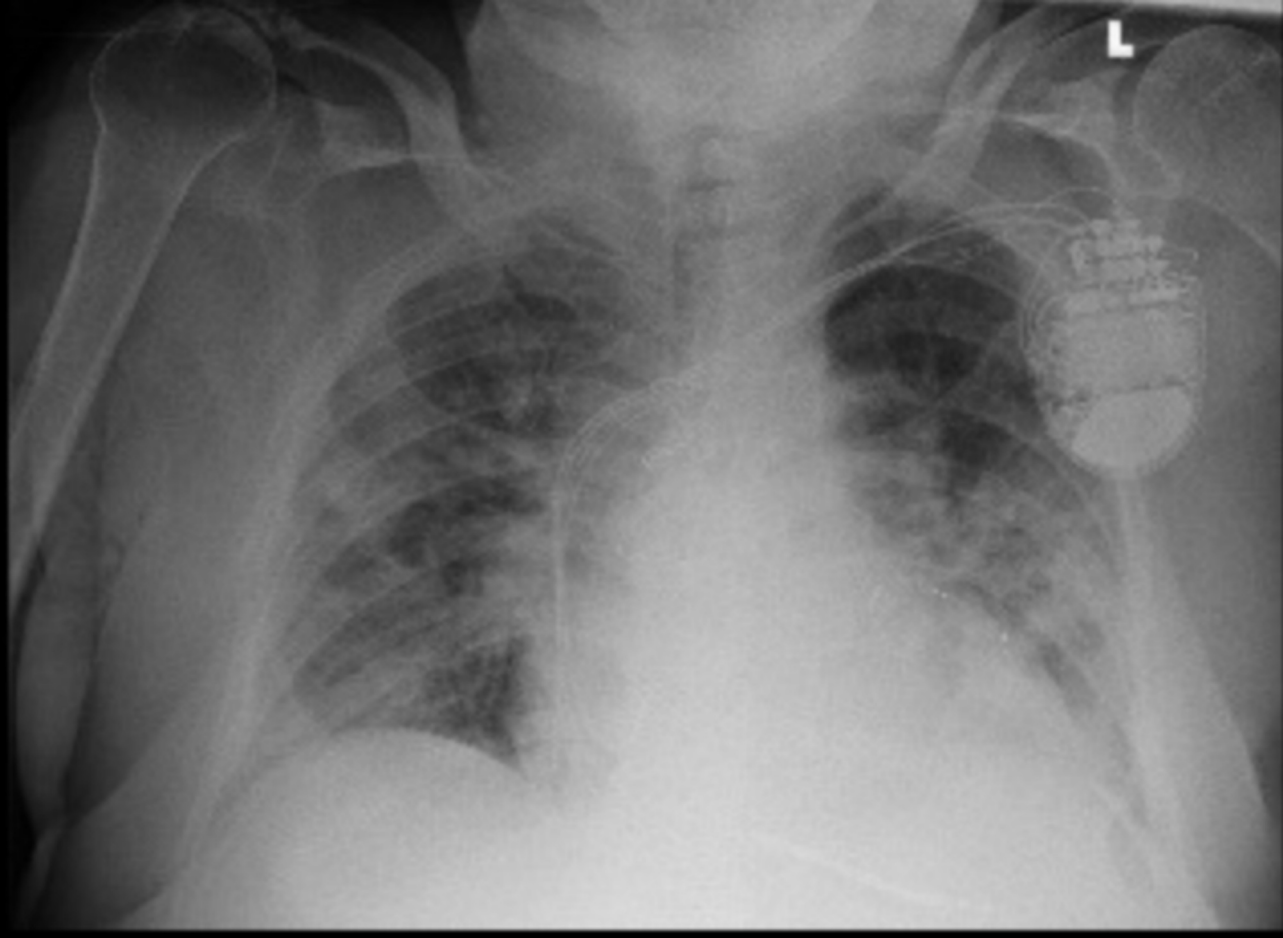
Day 4: portable chest X-ray showing worsening bilateral interstitial infiltrates
